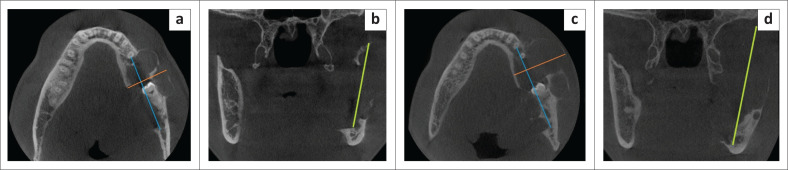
Size measurement of an ameloblastoma on cone-beam computed tomography at initial presentation (a, b) and after 11 months (c, d). The blue line represents the anterior-posterior dimension, the green line the superior-inferior dimension, and the orange line the medial-lateral dimension.
